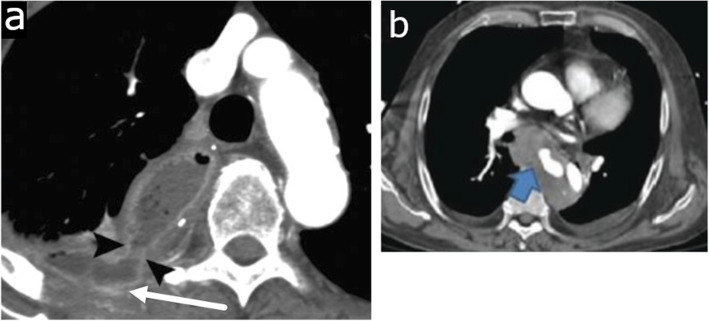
Esophageal fistulas. a Esophago-pleural fistula in a 61-year-old male with squamous cell carcinoma of mid esophagus, status post esophagectomy and gastric pull through presenting with fever. Axial CT image obtained 12 days after surgery shows a fistula (arrowheads) between the gastric tube and the right pleural space. Fluid collection with pleural enhancement and thickening (arrow) is suspicious for empyema. Reprinted with permission from Kim et al. Radiographics 2007; 27(2):409-429. b Aorto-esophageal fistula in a 70-year-old male patient with hypertension and regular alcohol use presenting with hematemesis and melena. Axial image of CT chest angiography shows type B aortic dissection with rupture, communication, and extravasation between aorta and esophagus (blue arrow) in the arterial phase. Reprinted from Kokatnur. Indian J Crit Care Med. 2015;19(2):119–121
